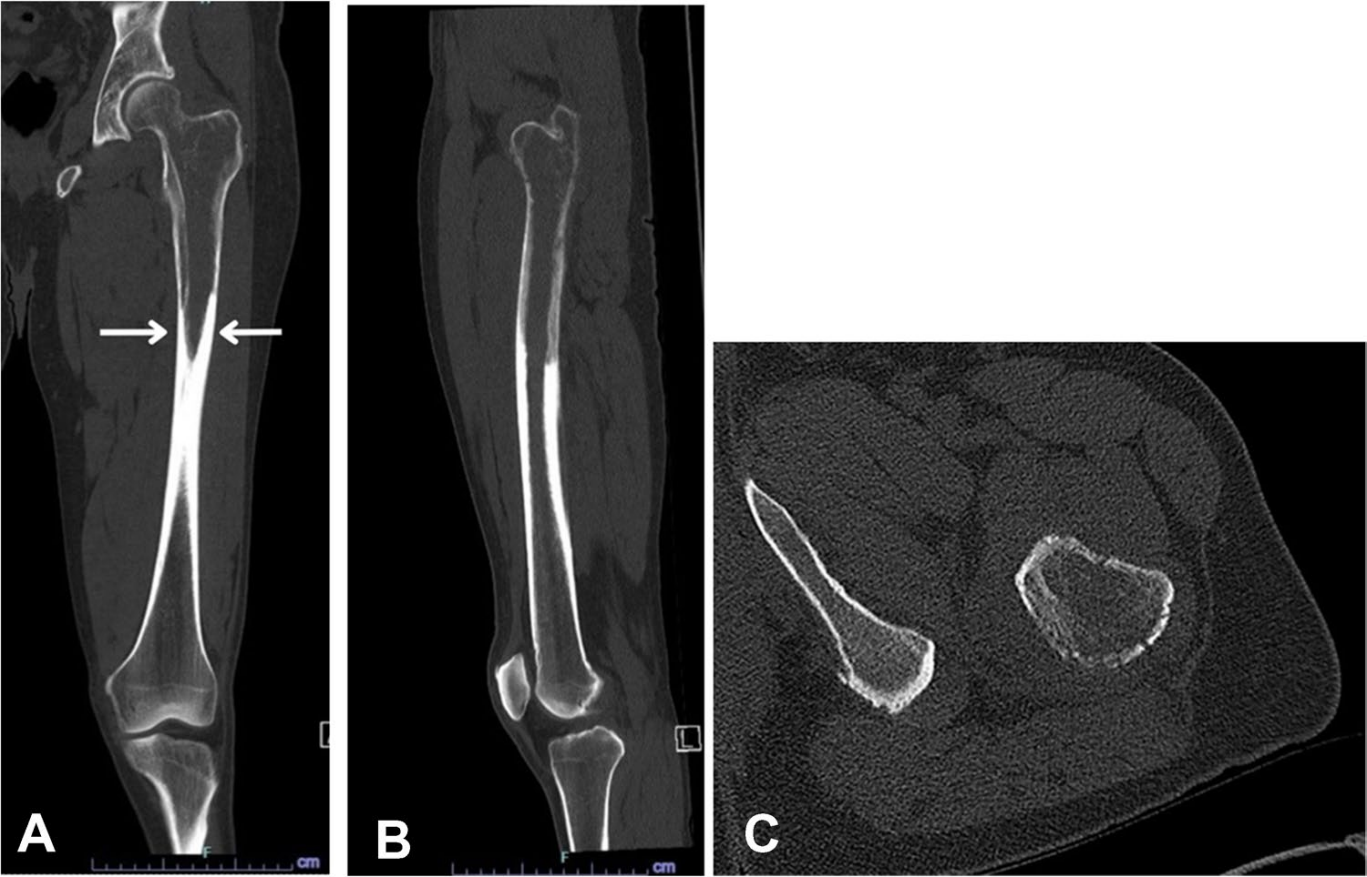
Coronal (A) and sagittal (B) CT images show cortical thickening with intracortical lucency and flame shape along the proximal diaphysis (arrows) and axial CT (C) also reveals the osteolytic lesion with permeative loss of definition of the surrounding cortex
